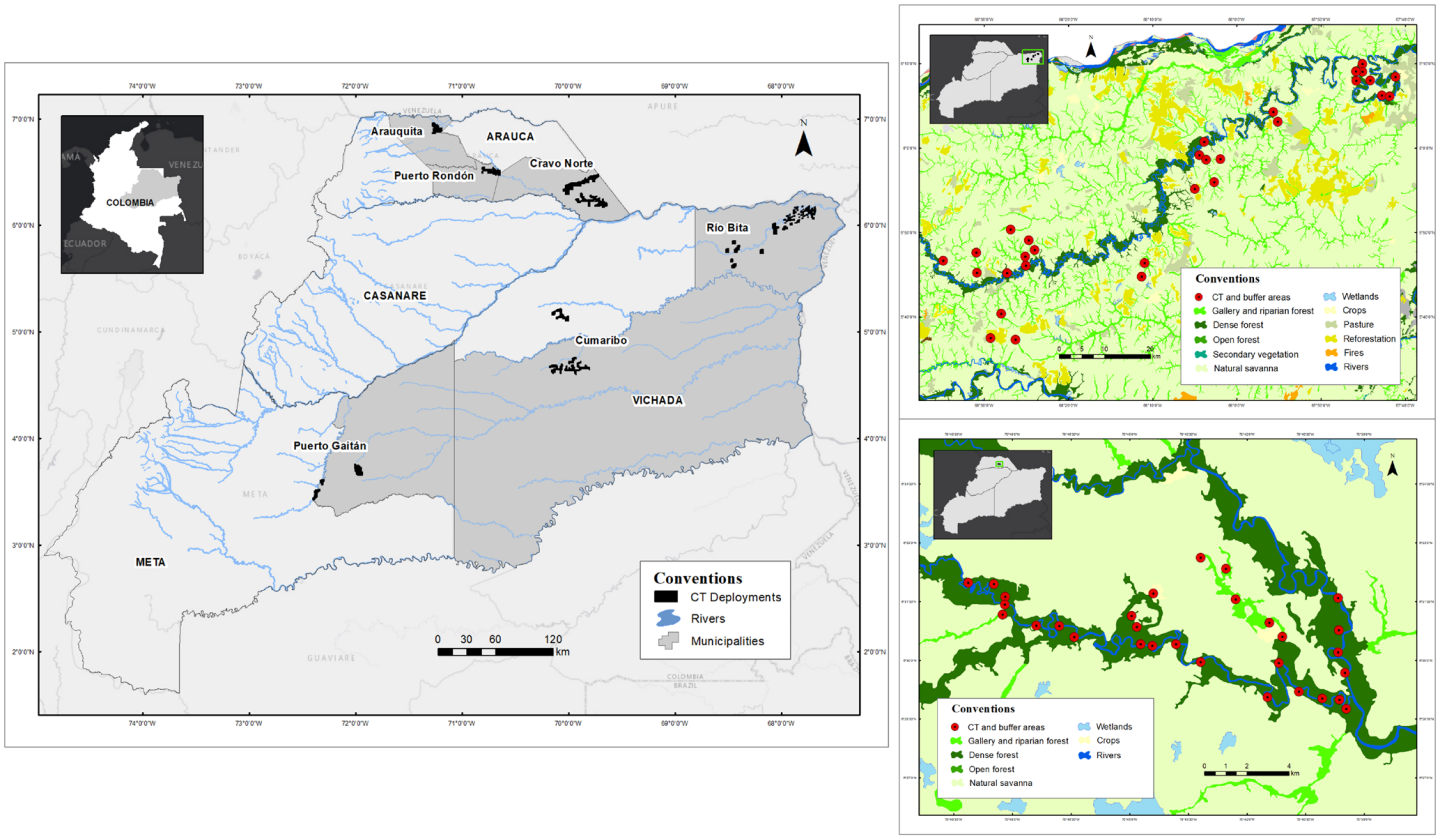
Study area in the Orinoquia region of Colombia showing the nine‐camera trap (CT) deployments used to estimate occupancy and detectability of the lowland tapir. The left panel highlights the general location of all deployments across Arauca, Casanare, Meta, and Vichada. The right panels show detailed land‐cover maps for two representative sites: (top) Puerto Rondón (Arauca) and (bottom) Río Bita (Vichada). Red circles indicate CT stations, each surrounded by a 1‐km buffer.
